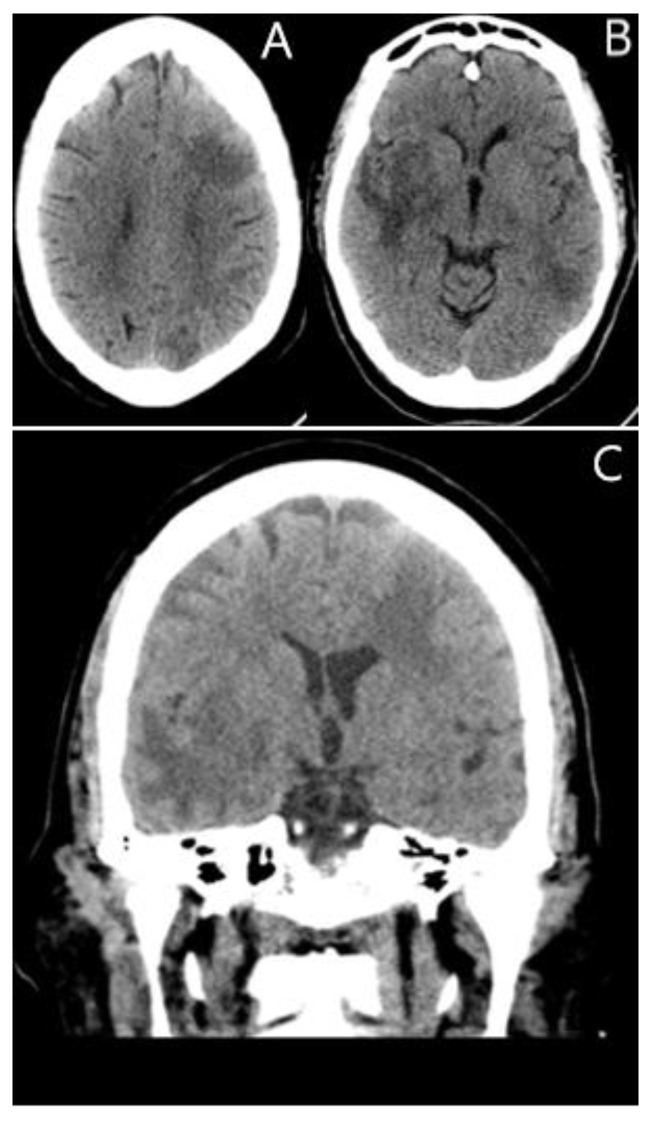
(A–C) Unenhanced Axial and Coronal CT images demonstrating vasogenic edema with a mass effect within the left frontal and bilateral temporal lobes. There is mild compression of the left lateral ventricle secondary to a left frontal lesion (C).
